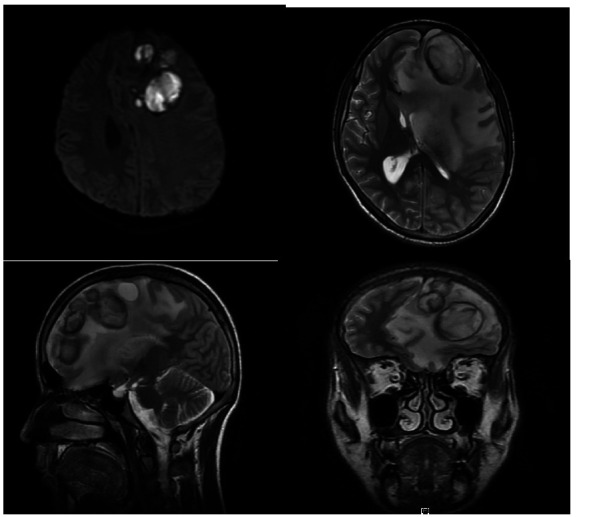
Magnetic resonance imaging in different planes showing multiple cerebral abscesses
